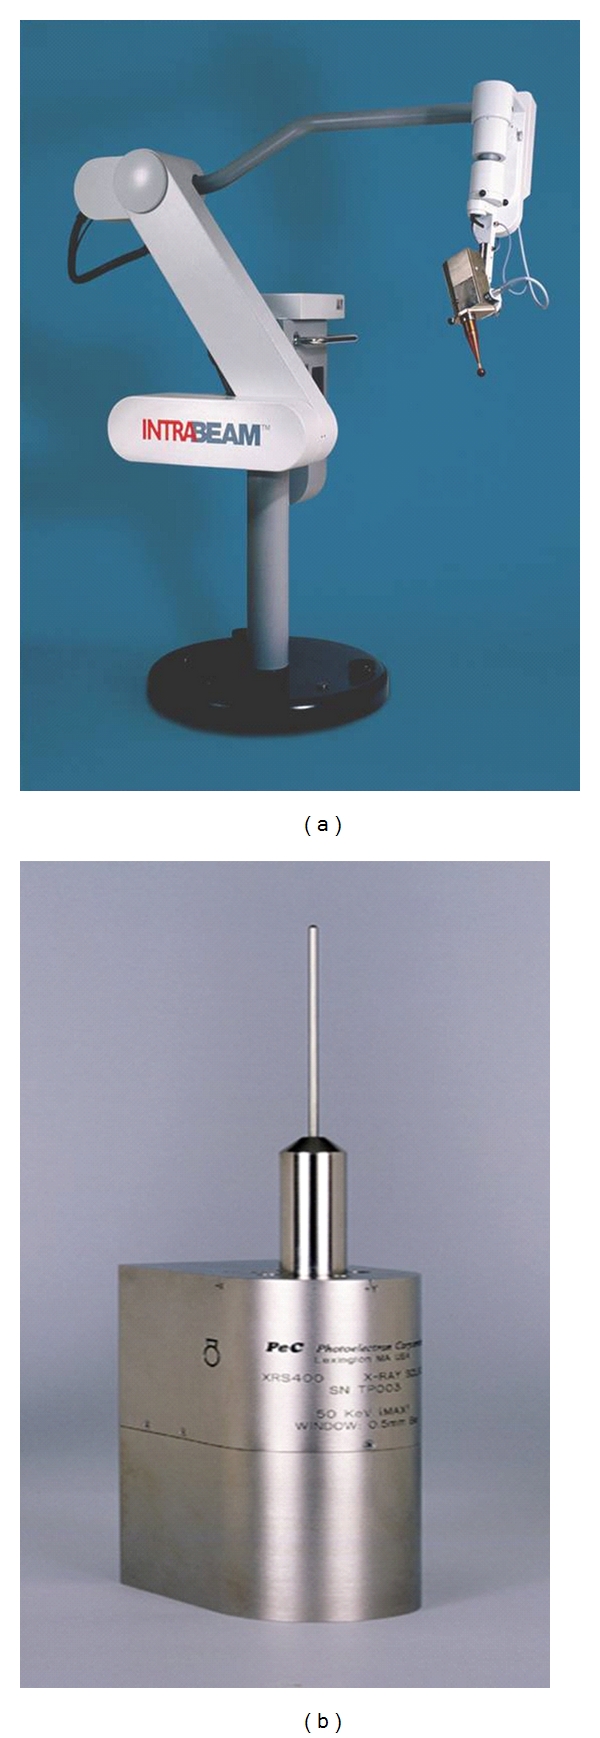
The Intrabeam System (a) and X-ray source (b).
